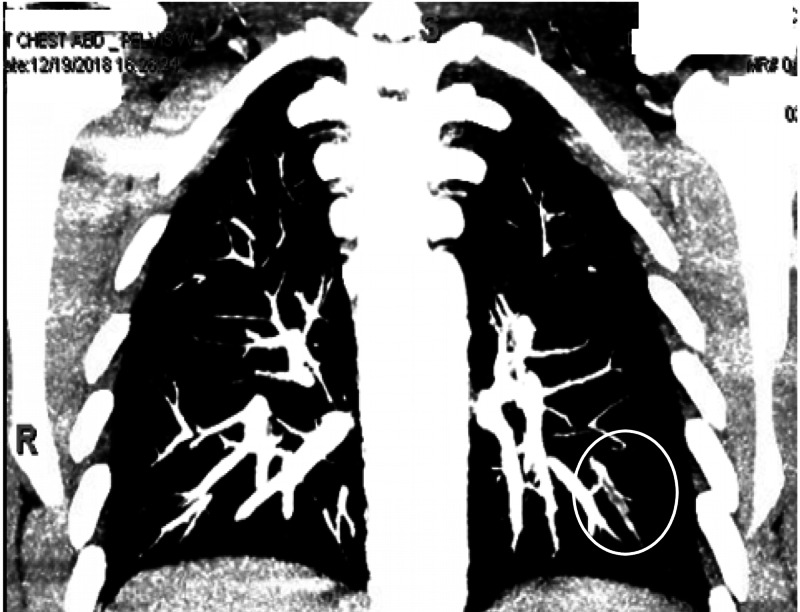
CT scan of the chest with contrast (coronal view) showing a filling defect in the left lower lobe segmental and subsegmental pulmonary arteries consistent with pulmonary embolism.
